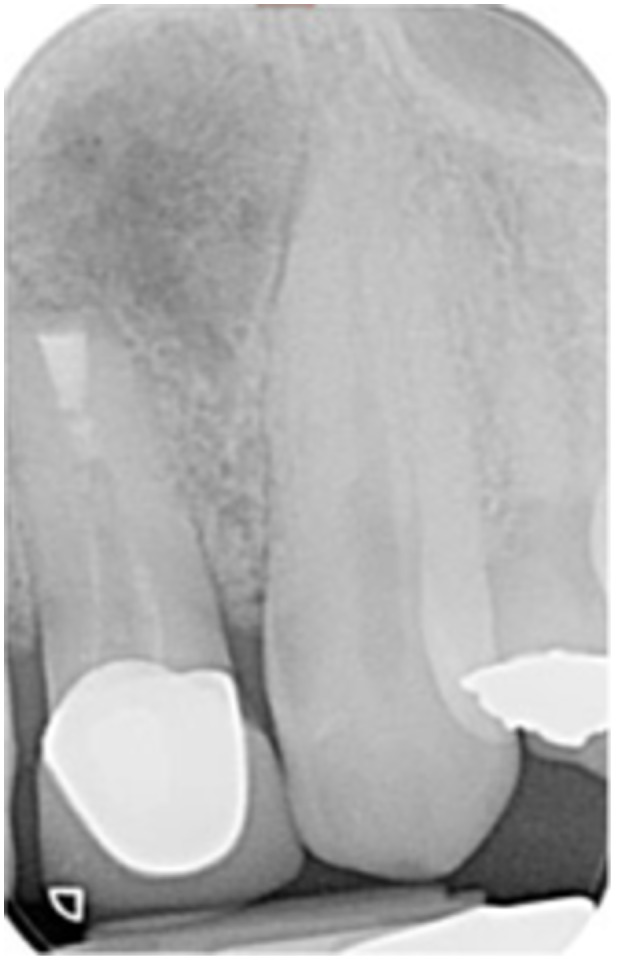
Case 10: The preoperative CBCT and periapical radiograph reveal a large periapical radiolucency associated with tooth 22, which has thinned the labial cortical plate. Apical surgery was performed on tooth 22 without prior nonsurgical root canal retreatment due to the presence of a fiber post, complicating the nonsurgical root canal retreatment. Nonetheless, the crown had satisfactory margins. At the 6‐month follow‐up, a significant reduction in the size of the periapical radiolucency can be seen.
